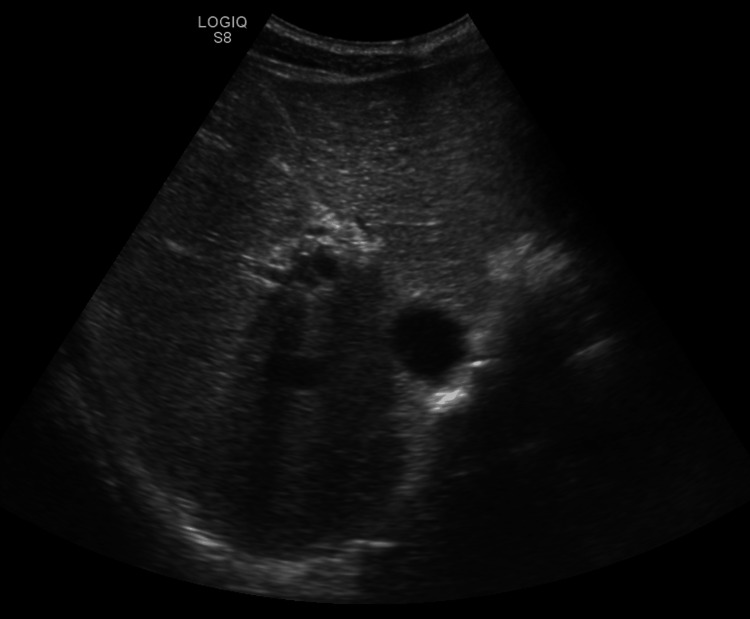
Abdominal ultrasound showing normal-sized liver with regular contours, showing mild steatosis and multiple infra- and juxta-centimetric cystic images scattered throughout both lobes.
